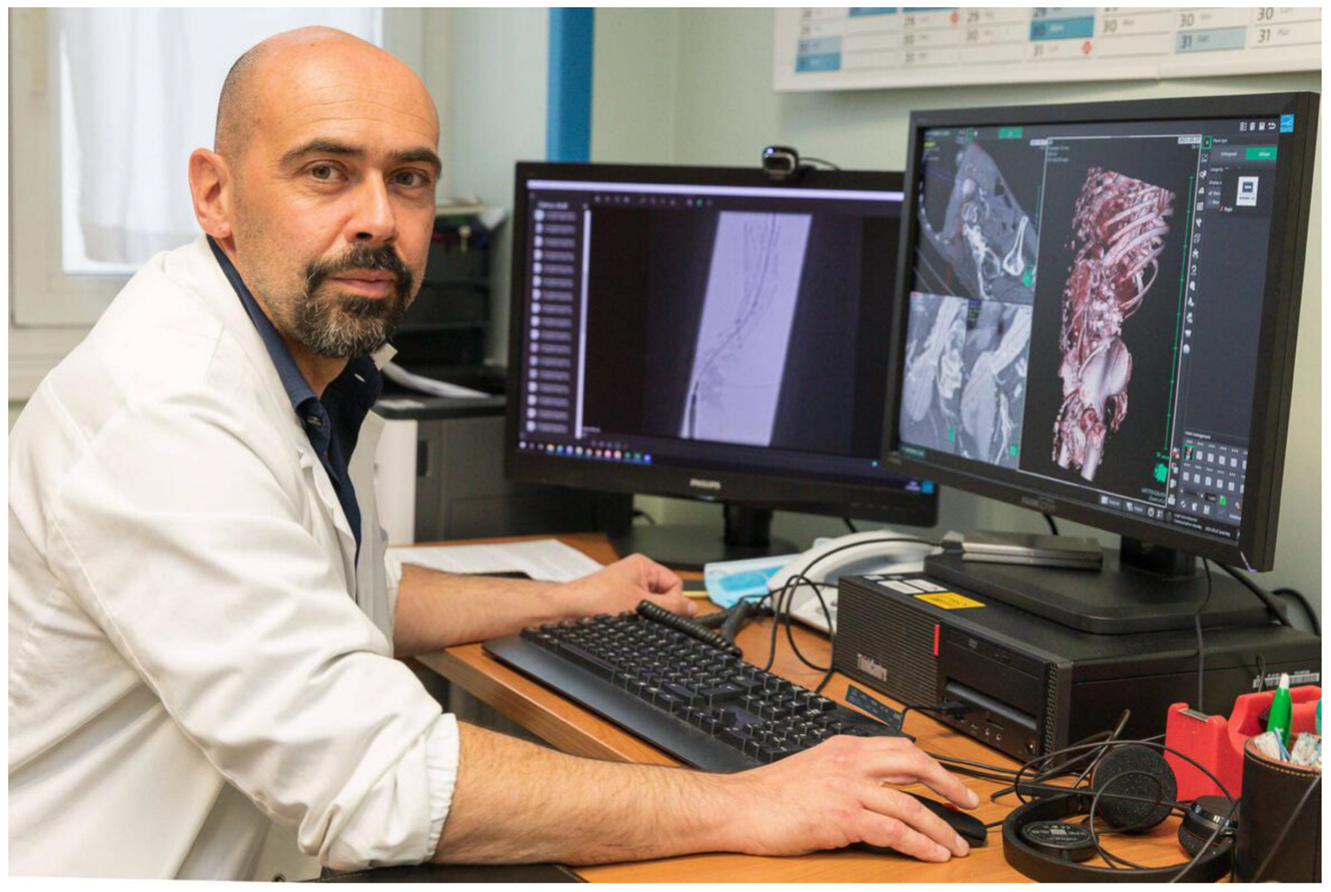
Stefano Bonvini with a comparison between the raw models as extracted from CT data and the final model ready for printing (
